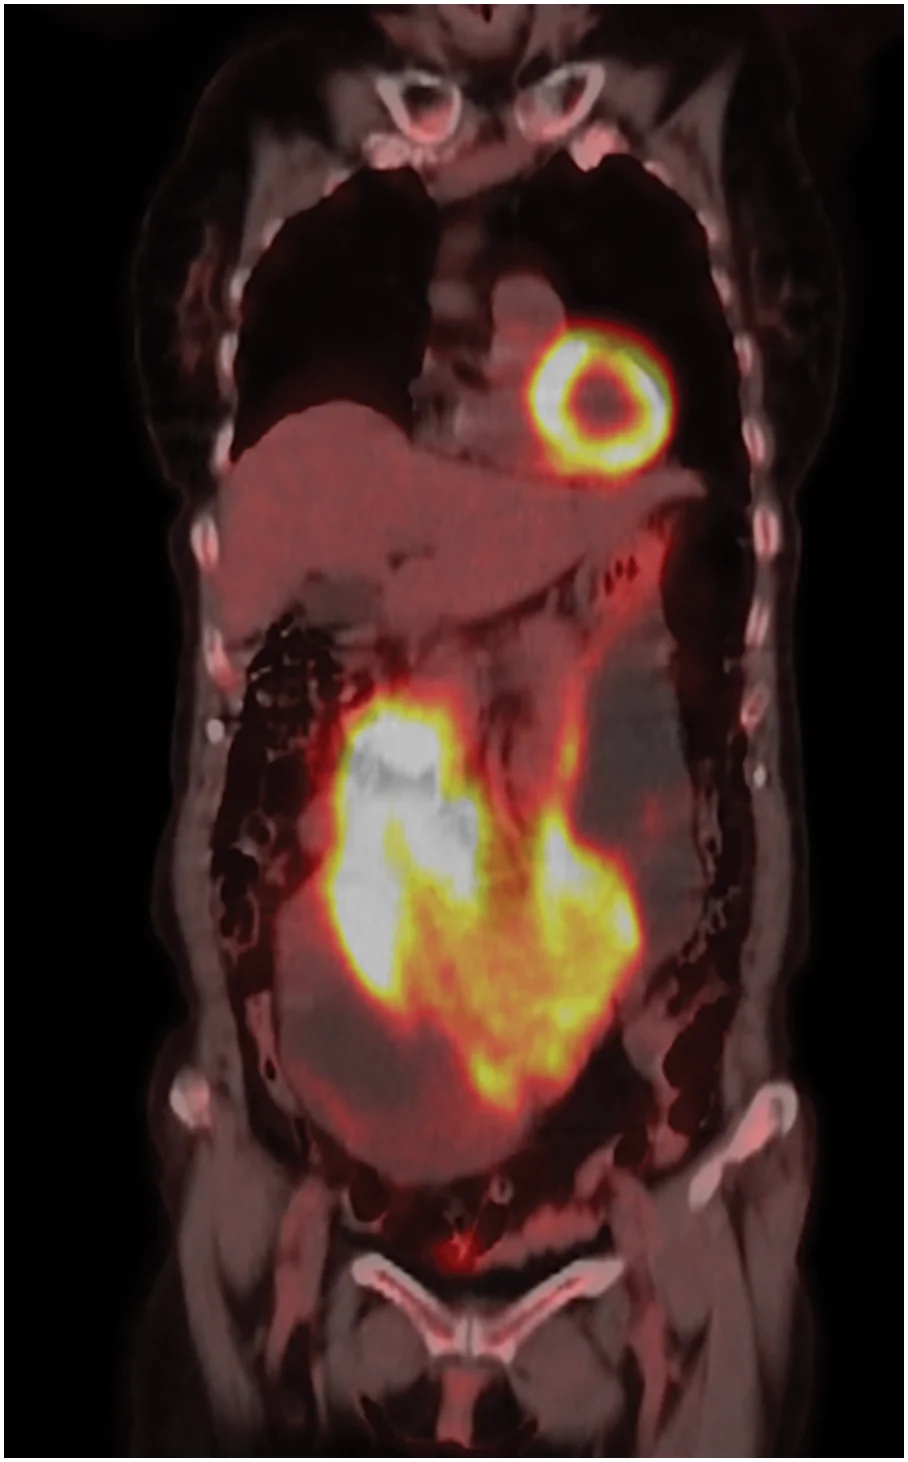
PET-CT findings of the tumor. PET–CT shows increased fluorodeoxyglucose uptake within the intra-abdominal tumor, without lymph node or distant metastases.
